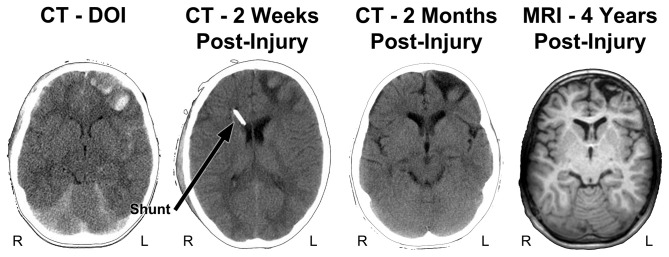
Parenchymal changes over time from the DOI scan to 4 years post-injury. The DOI through the scan at 2 months are all from CT and the far right image is a T1-weighted MRI, at 4 years post-injury. In contrast to the CT images shown in Figure 3, the slice level of these images are all at the level of the anterior horn of the lateral ventricle. Note that where the most prominent acute hemorrhages and frontal contusions occur on the DOI scan that this also becomes the center-point for tissue dissolution (encephalomalacia) over time. Shortly after the DOI scan was obtained the child received an intraventricular shunt to aid in intracranial pressure reduction and monitoring. By 2 months post-injury the focal encephalomalacia is very prominent (white arrow) and there is also prominence of the Sylvian fissure (black asterisk) along with ventricular enlargement, indicative of some generalized cerebral volume loss. R, right; L, left.
